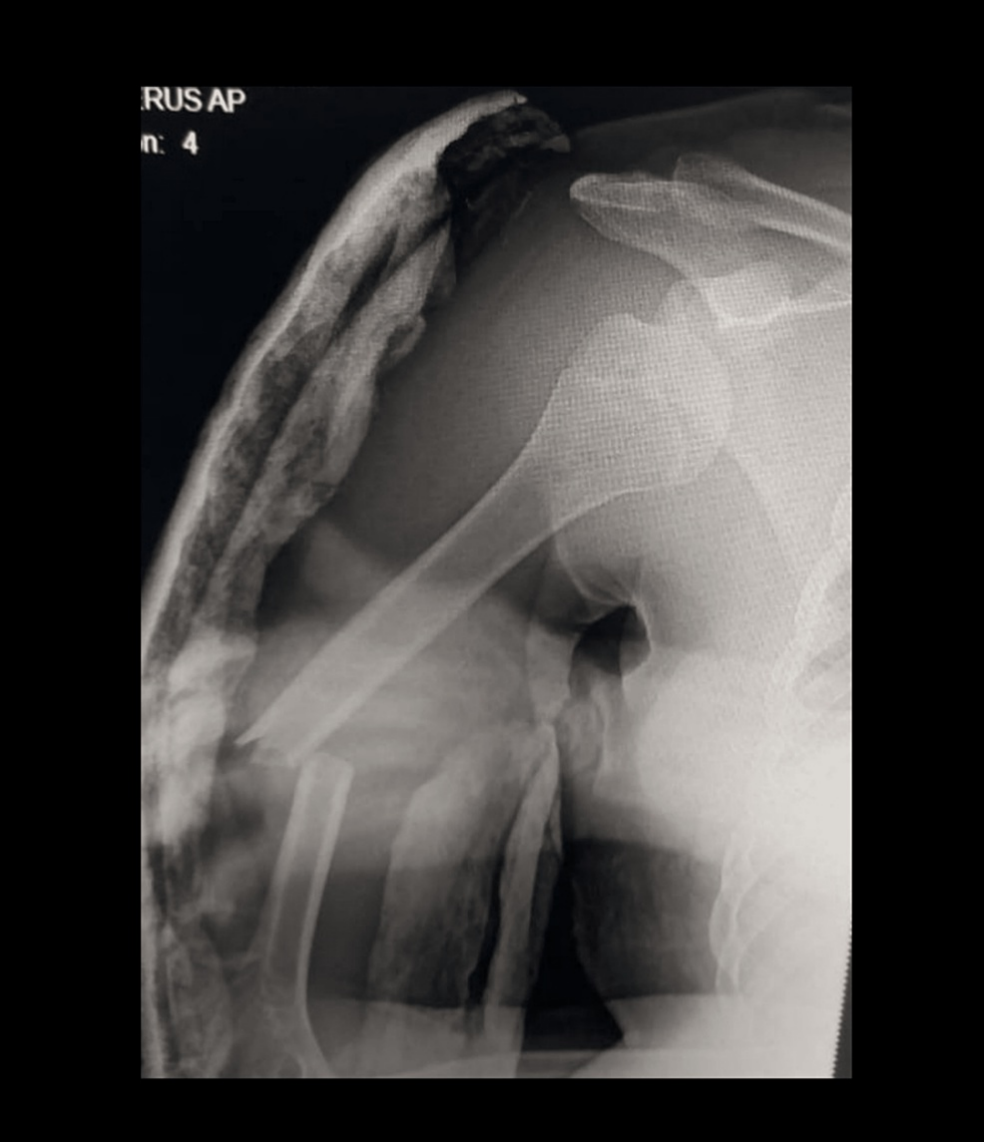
X-ray showing anterior dislocation of the right shoulder with an ipsilateral mid-shaft fracture of the humerus
